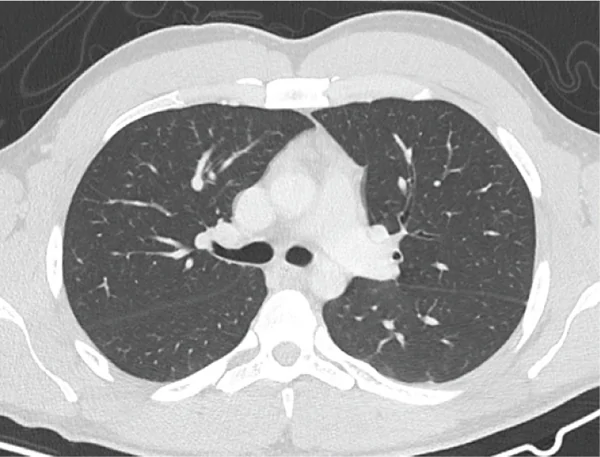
Initial chest computed tomography demonstrating normal lung parenchyma, without consolidations, ground‐glass opacities, or pleural effusion.
